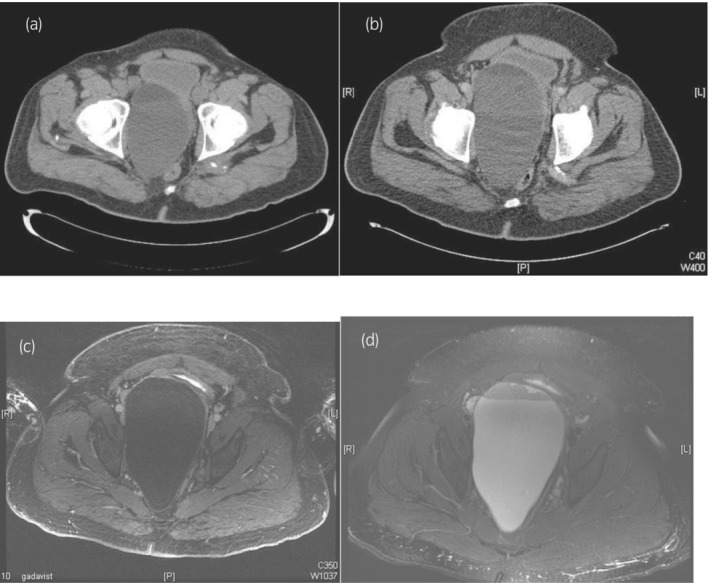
Axial images of the patient's tumor 4 years after having an aspiration of a possible abscess from his right seminal vesicle cyst. In (a) the mass measures 12.7 × 8.9 cm. In (b) 8 years after the initial presentation, the mass increases in size and measures 15.3 × 9.8 × 16.7 cm with obvious mass effect on the bladder. Finally, prior to presentation to a urologist, a MRI scan with gadolinium was done which shows a 17.7 × 12.0 × 11.1 cm mass that in (c) that is non‐enhancing in comparison the bladder which enhances anteriorly in the T1 phase and fat filled in the T2 phase as shown in (d).
